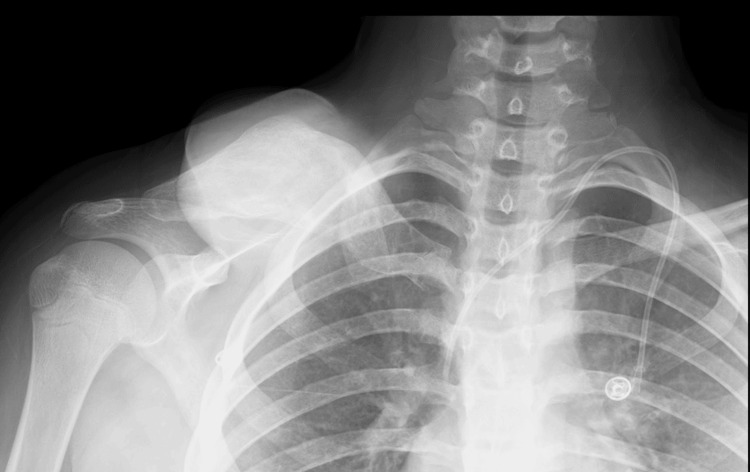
Chest X-ray of the patient before chemotherapy The images were taken from the patient's electronic records after securing consent from the patient for the publication, reproduction, broadcast, and other use of imaging and photos.
